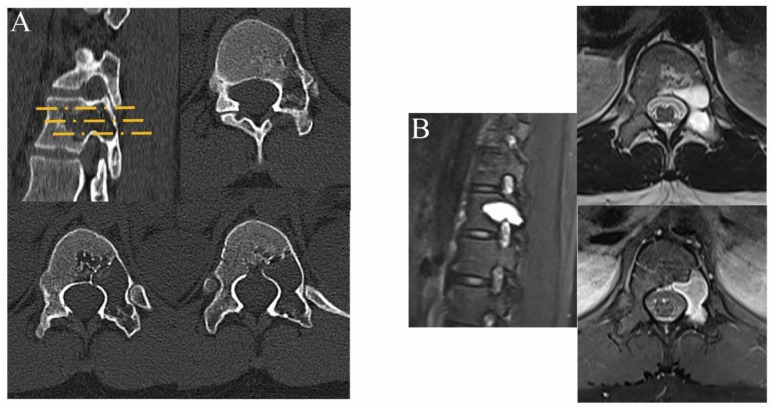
(A) CT scanning showed that part of the vertebral body and pedicle on the left side of the T12 vertebral body showed slight expansive bone destruction, the marginal bone was slightly sclerotic, the boundary was clear, and there was no periosteal reaction. (B) MRI images showed cystic high signal shadows in the left part of the T12 vertebral body and in the pedicle of the vertebral arch, which could be evenly enhanced so no soft tissue mass shadow was seen and the paravertebral space was clear.
